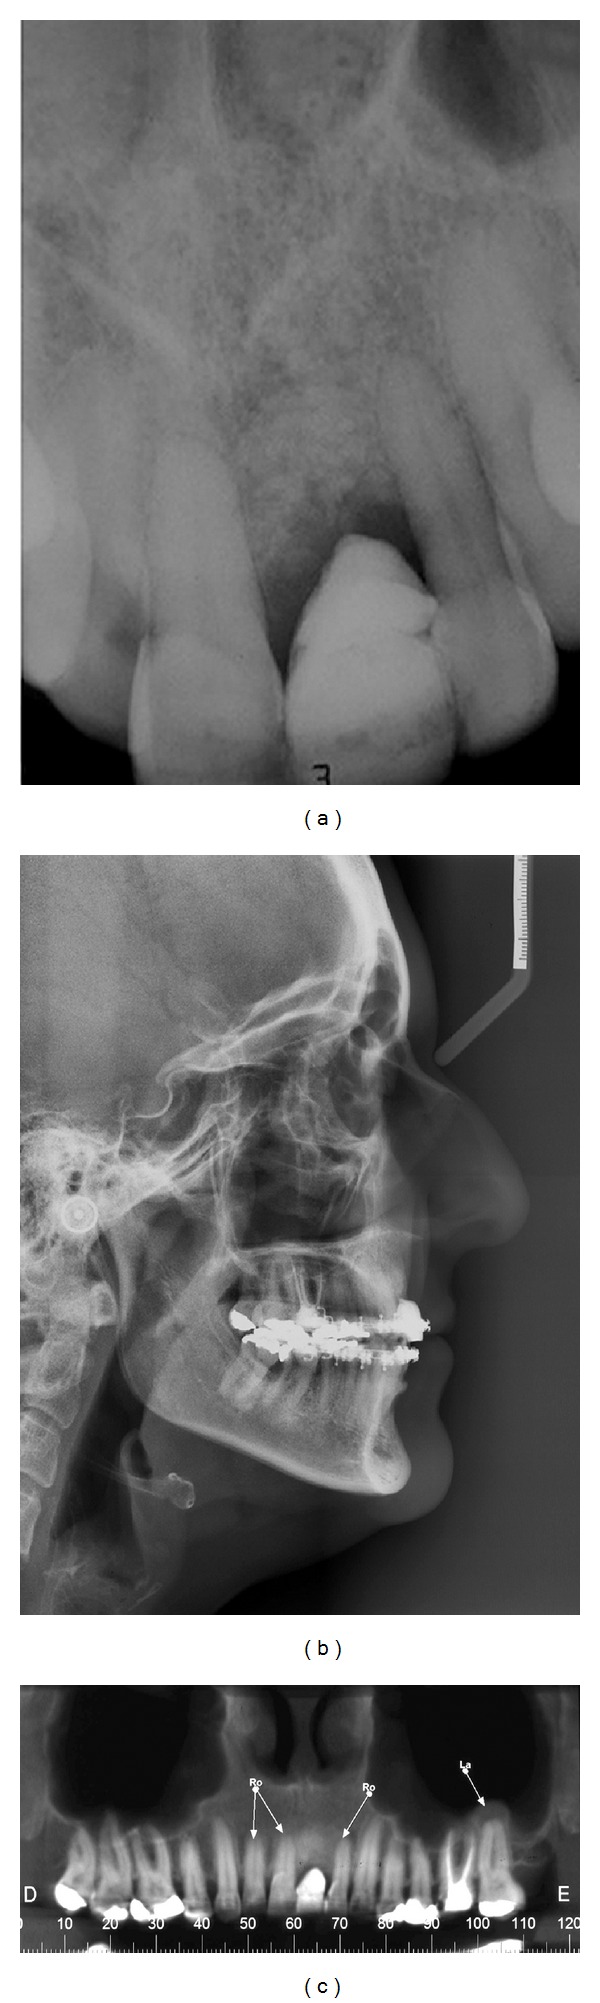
Periapical, cephalometric, and panoramic radiography after orthodontic movement showing alveolar bone augmentation allowing implant placement.
